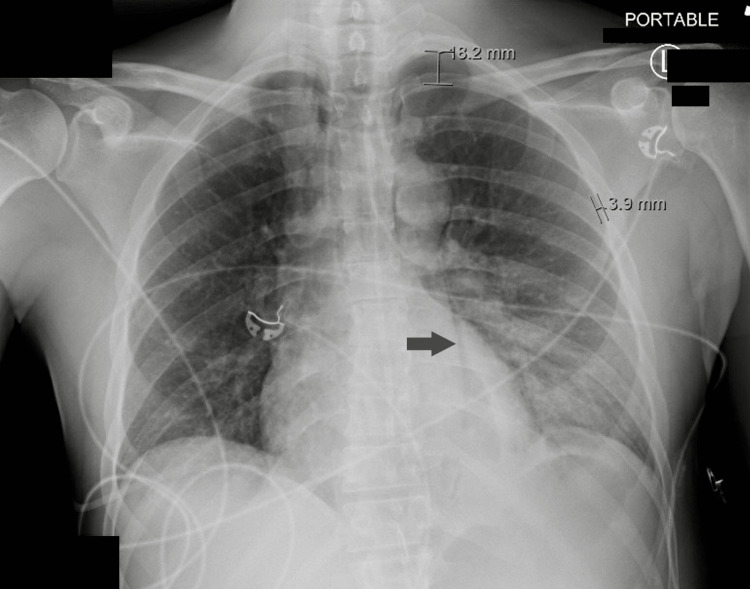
AP portable chest x-ray showing pneumothorax and mediastinal air (arrow) AP: anterior-posterior.
